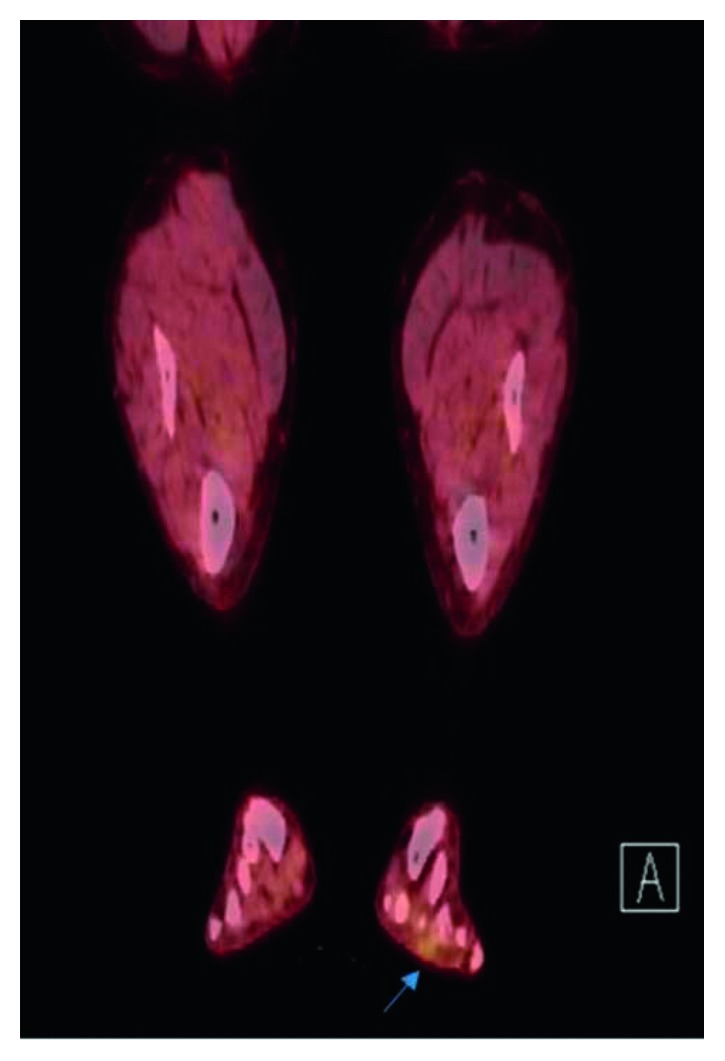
Positron emission tomographic image of the lower extremities showcasing a fluorodeoxyglucose (FDG) avid spot on the plantar surface of the left foot (arrow).
